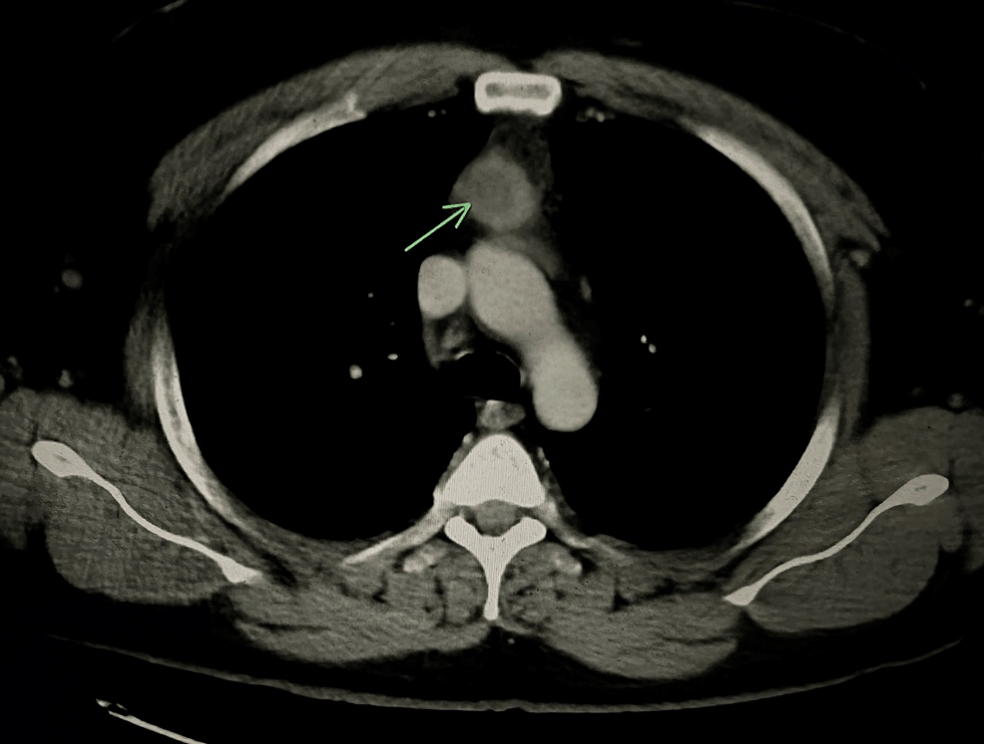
Axial CT image of the chest showing the anterior mediastinal mass CT: computed tomography
